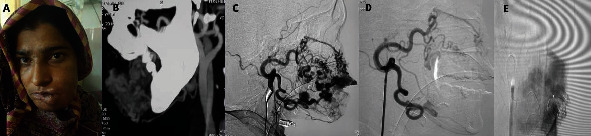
A 45-year-old female with insignificant medical history presented with progressively increasing swelling involving, upper lip, left cheek, and left side of the mandible. (A) Preembolization photograph showing extensive growth involving left lower half of face and lips. (B) Computed tomographic angiographic reformat of the left mandible area showing enlarged vascular channels. (C) Selective left external carotid artery angiogram, lateral projection, showing AVM feeding by linguofacial trunk and internal maxillary artery. (D) Postembolization, left external carotid artery angiogram showing significant reduction in the flow of nidus. (E) Superselective angiogram with microcatheter during embolization showing squid cast.
